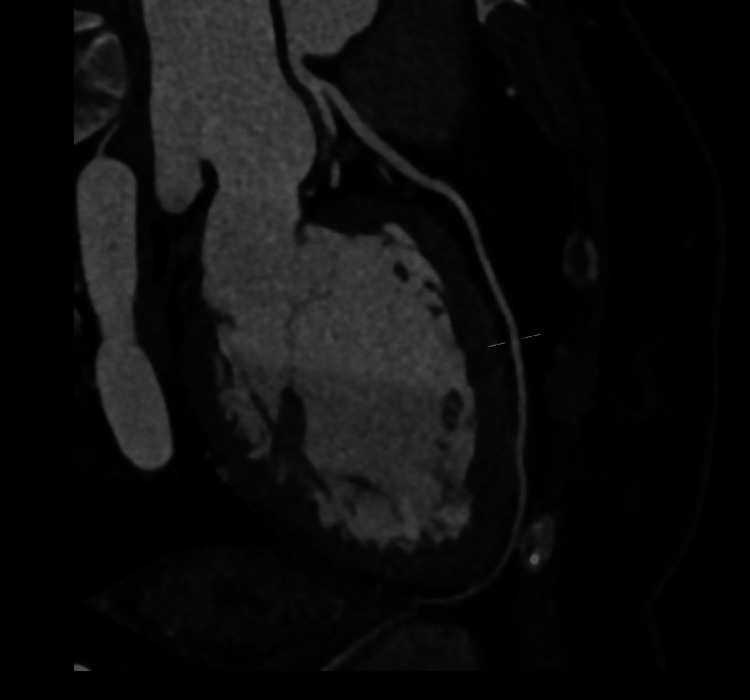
CT coronary angiogram showing patent left anterior descending artery
